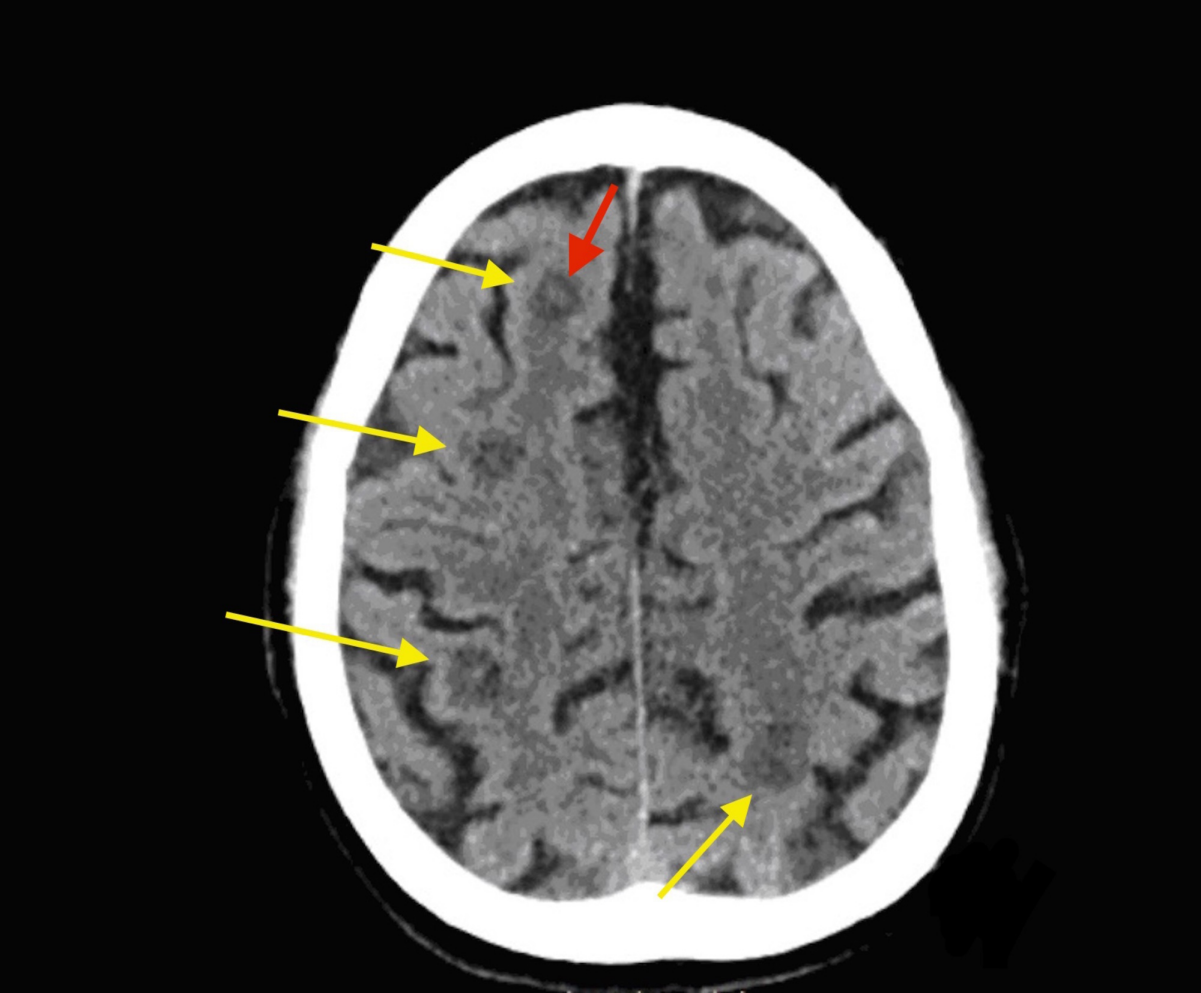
Computed tomography (CT) scan of the head showing hypointense lesions in the fronto-parietal region (yellow arrows). There is hyperintense signal in the center of this lesion consistent with hemorrhage (red arrow).
